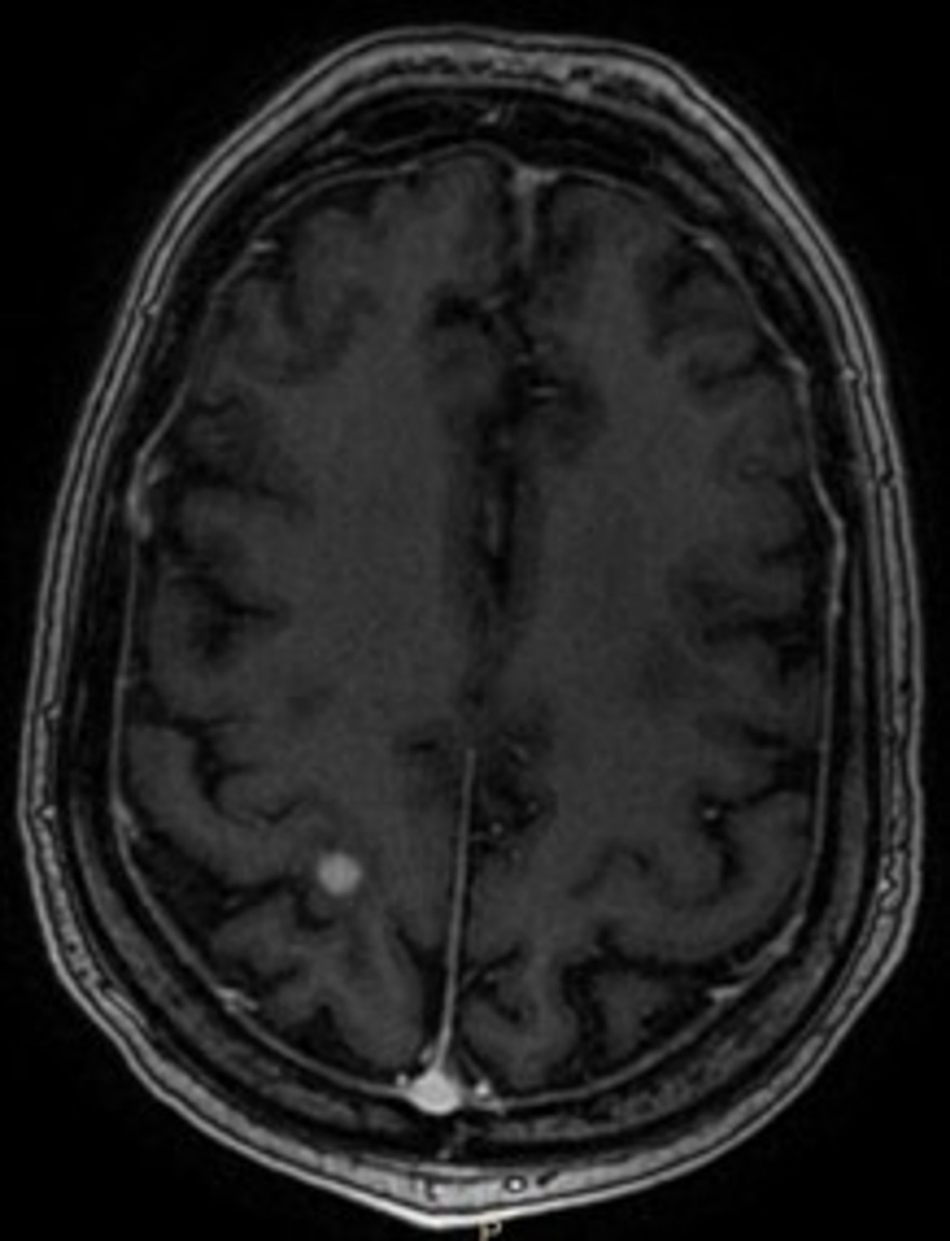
MRI brain with contrast, T1-weighted image showing 0.7 cm high right parietal lobe lesion
